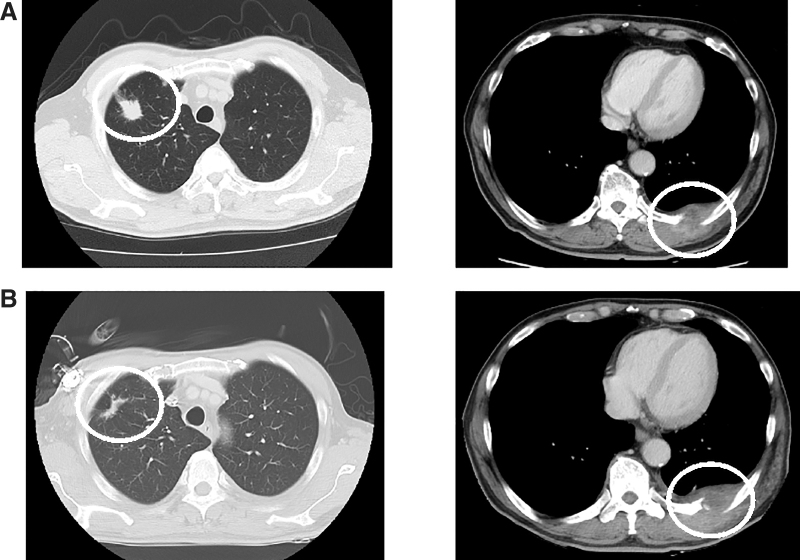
Chest computed tomography. (A) Tumors are found in the right lung field and left chest wall. (B) The tumor in the left chest wall is slightly enlarged. The red circles indicate the locations of the tumors.
